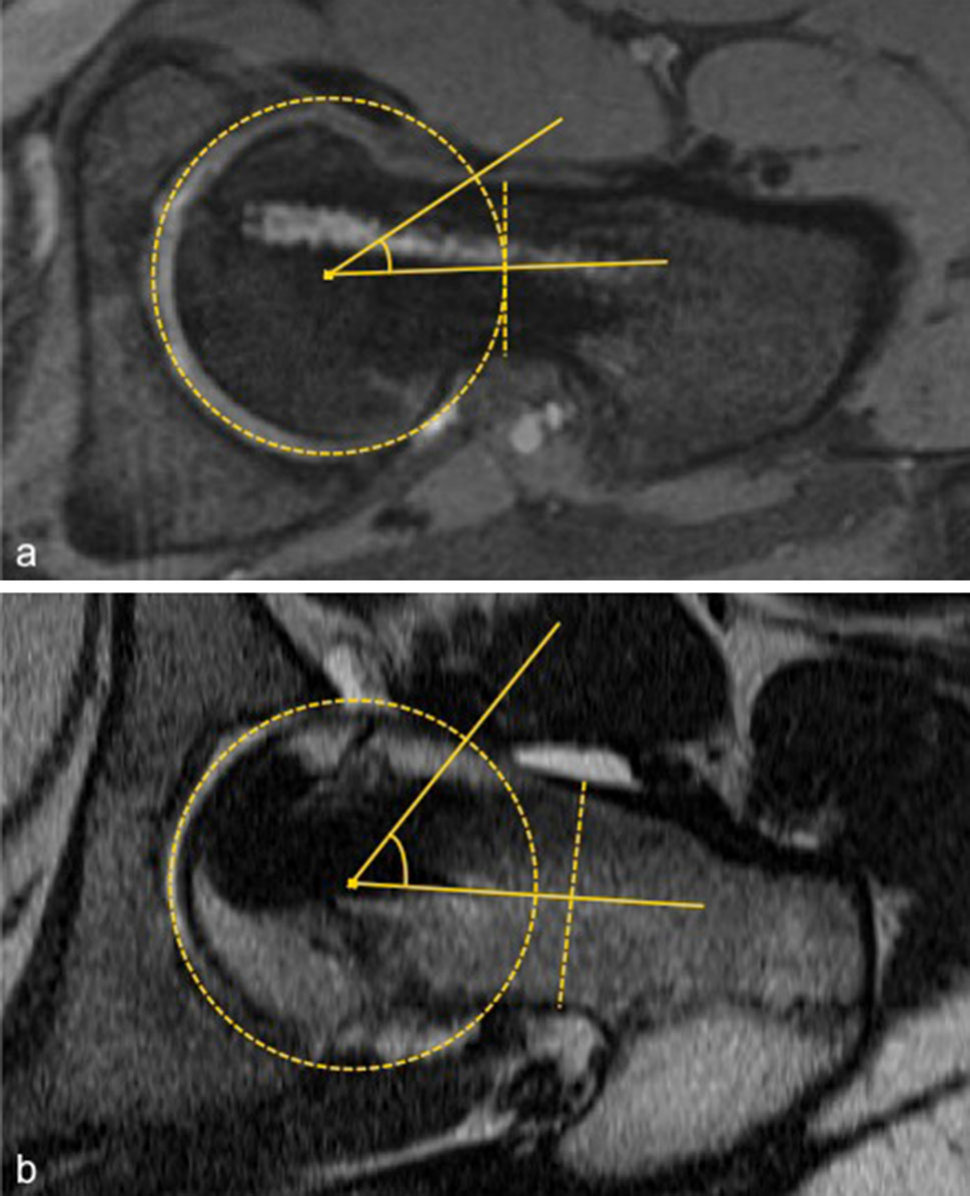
Alpha-angle on radial reformatted MR images (patient no. 1) a prior and b after hip arthroscopy [8, 19]
